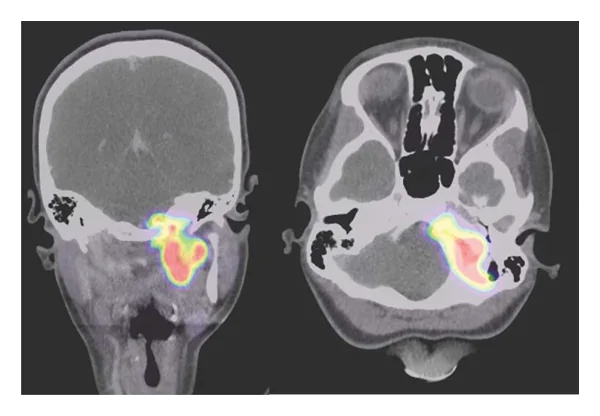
Pretreatment DOTATATE PET‐CT showing an avid left paraganglioma.
